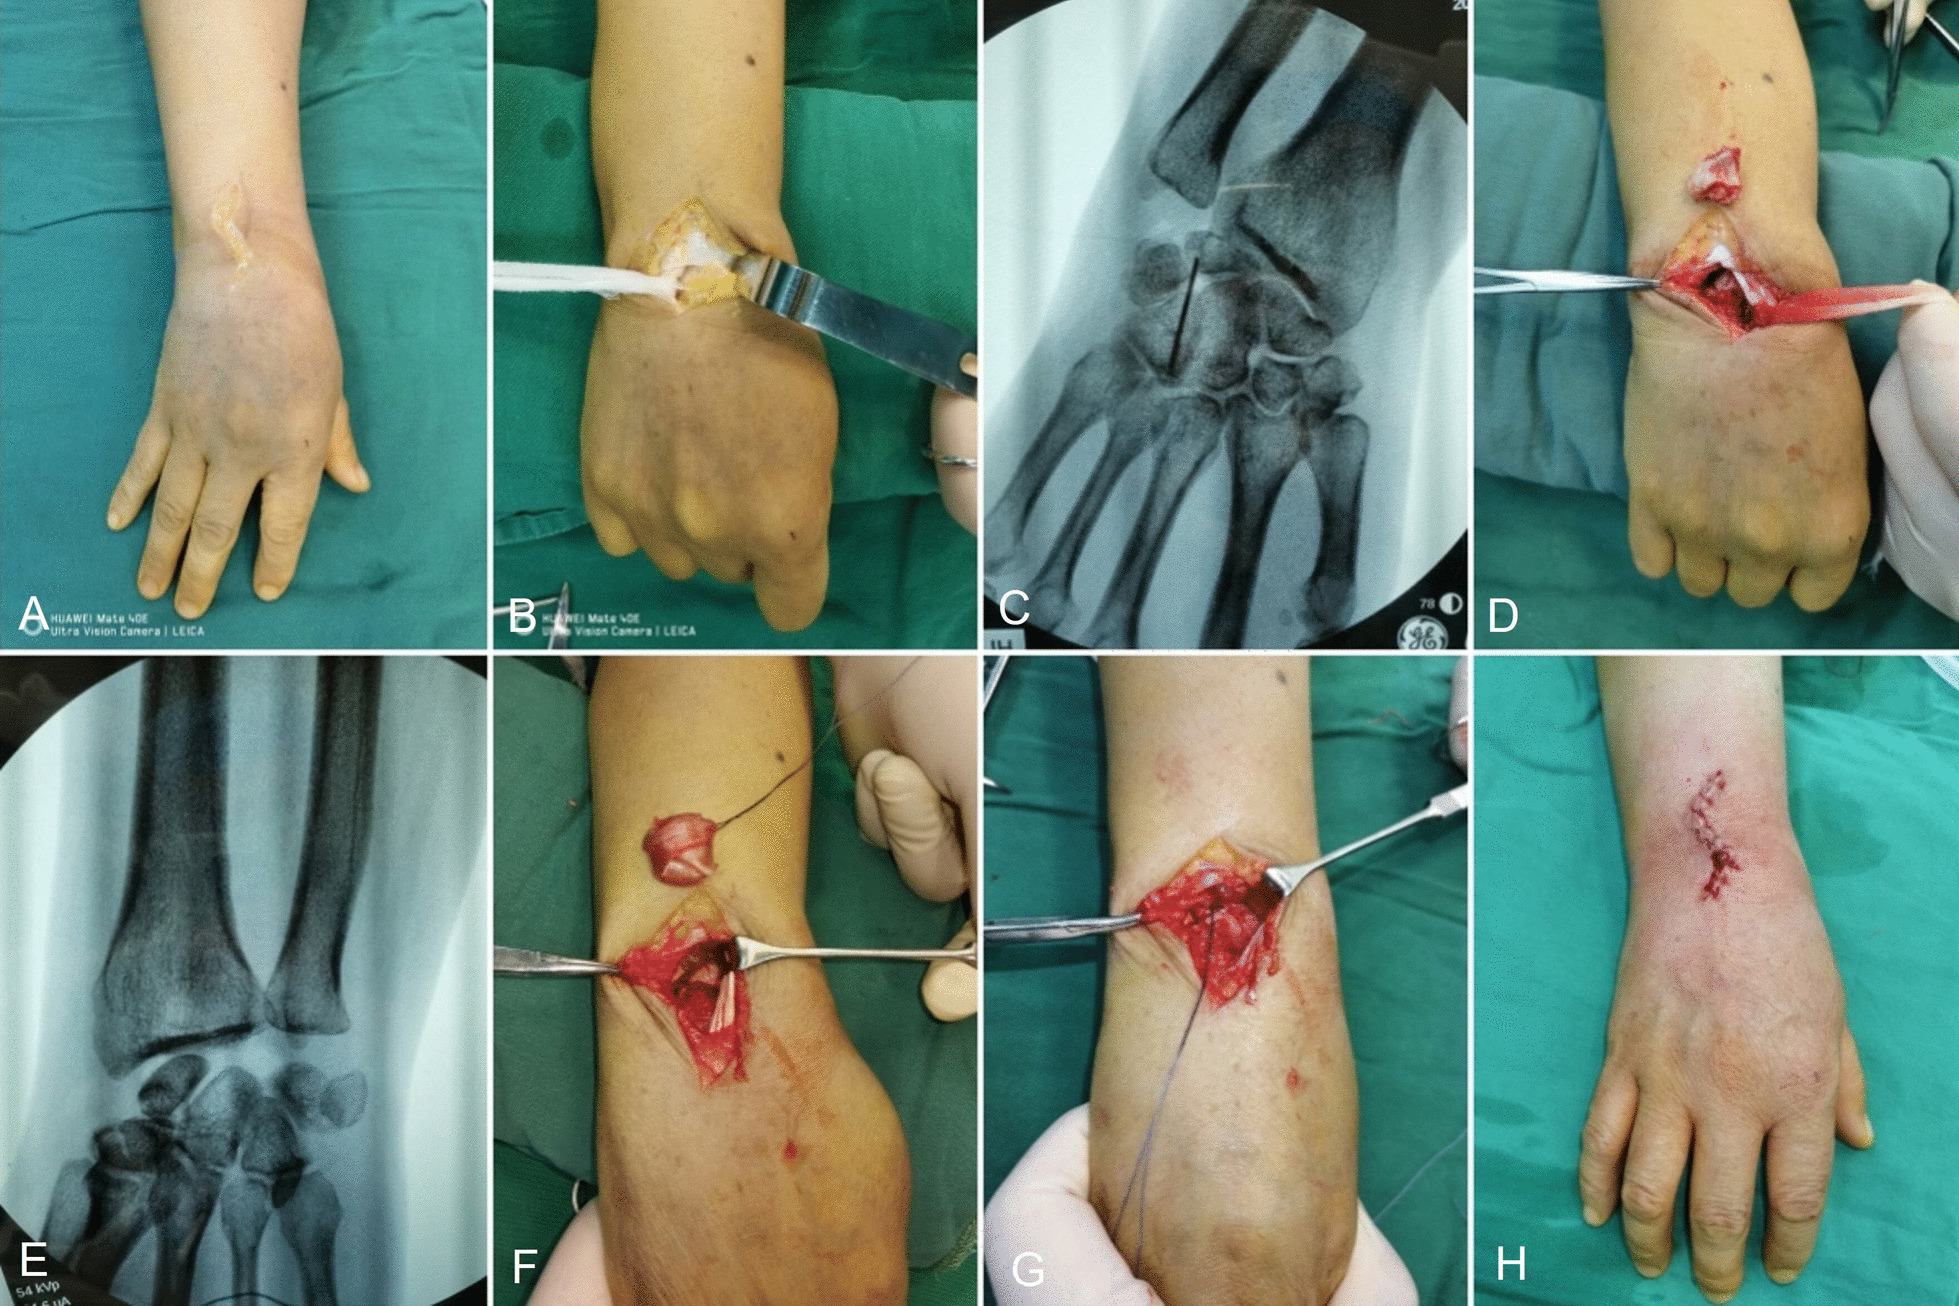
An S-shaped incision was made in the middle of the dorsal wrist (A); the extensor tendon was retracted to expose the dorsal wrist joint capsule (B); X-ray confirmed the position of the necrotic lunate (C); the necrotic lunate was removed, and the defect space was left (D); X-ray confirmed that the necrotic lunate was completely removed (E); the palmaris longus tendon ball was sutured into a ball (F); the palmaris longus tendon ball was placed in the defect space after lunate resection (G); and the joint capsule was repaired, and the incision was closed (H)
